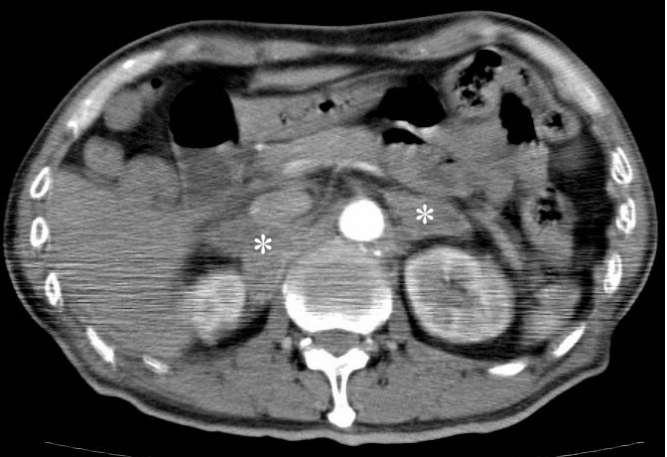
CT scan showing bilateral adrenal enlargement consistent with lung cancer metastases (asterisk).
